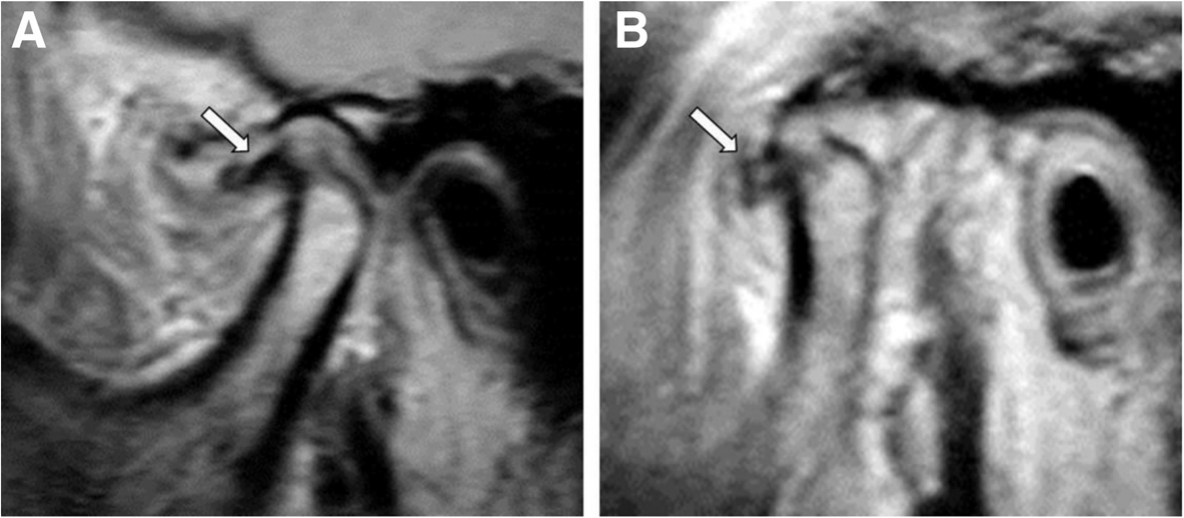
Representative MRI of ADD without reduction and an erosive condylar surface of TMJ. a and b The shape and length of the disc (*white arrows*) was also deformed in the closed and open mouth positions
